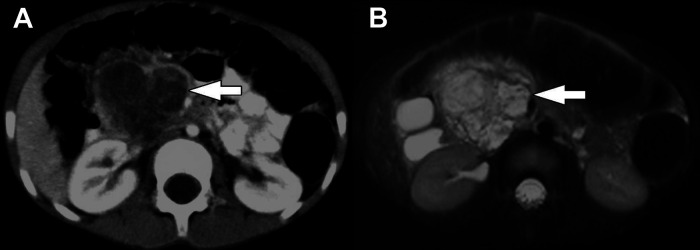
Pancreatoblastoma. (A) Axial CT of the abdomen obtained in the portal venous phase following intravenous contrast material administration shows a heterogeneously hypoenhancing mass arising from the head and neck of the pancreas (arrow). (B) T2-weighted fat-saturated MRI in the same patient shows the mass (arrow) to be heterogeneously hyperintense with the appearance of internal complexity.
